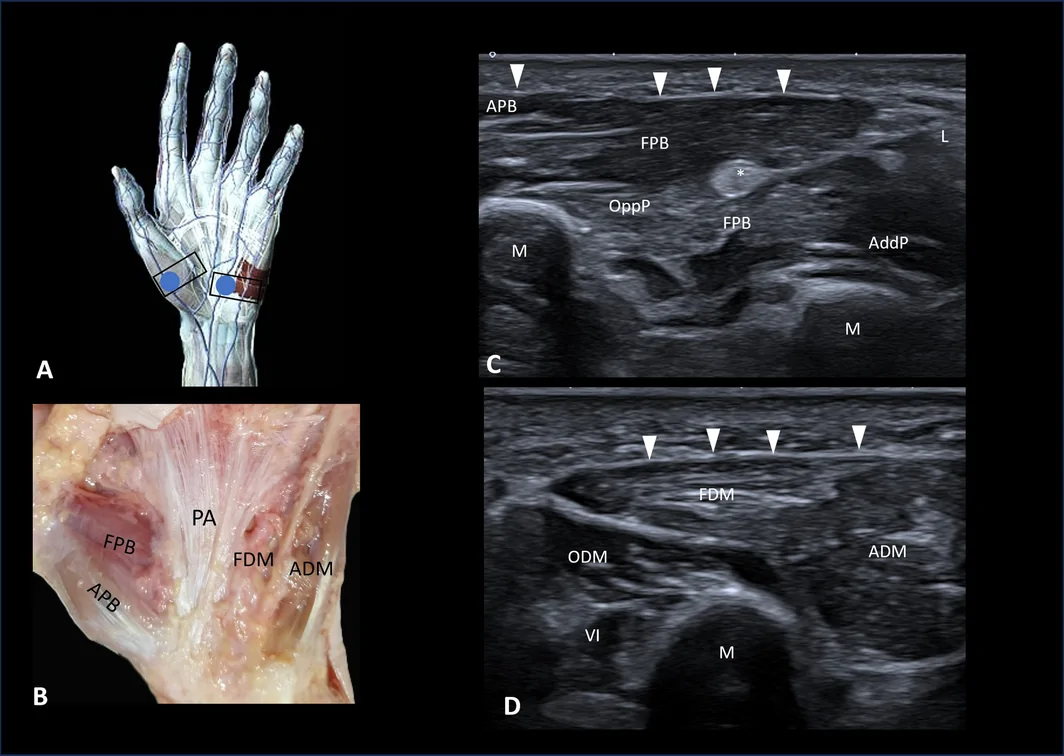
Thenar and hypothenar fasciae. A, Probe positioning. B, Anatomical dissection showing the thenar and hypothenar fasciae, appearing as epimysial layers, often less distinct from the underlying muscles. C,D, Ultrasound images revealing the thenar and hypothenar fasciae (arrowheads) as a thin hyperechoic band. APB, abductor pollicis brevis muscle; FPB, flexor pollicis brevis muscle; OppP, opponens pollicis muscle; L, lumbricalis muscle; AddP, adductor pollicis muscle; *, flexor pollicis longus tendon; FDM, flexor digiti minimi muscle; ADM, abductor digiti minimi muscle; VI, ventral interosseus muscle; M, metacarpal bone.
